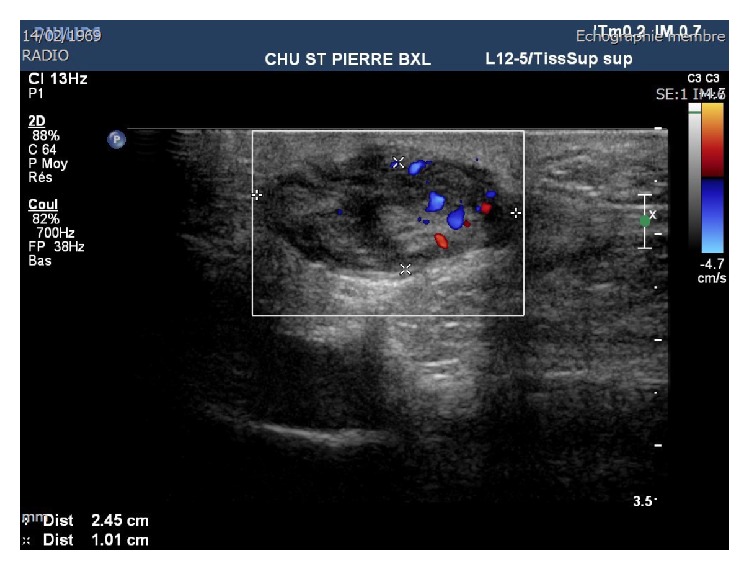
An ultrasound of the plantar arch was made and found a hypoechoic and homogeneous nodule at the thickness of the plantar fascia with a significant hyperemia in Doppler.
